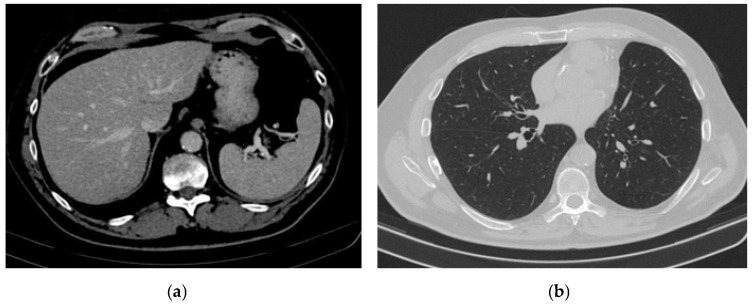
Complete remission. (a) Abdominal CT scan: complete remission of hepatic metastases (b) Chest CT scan: complete remission.
